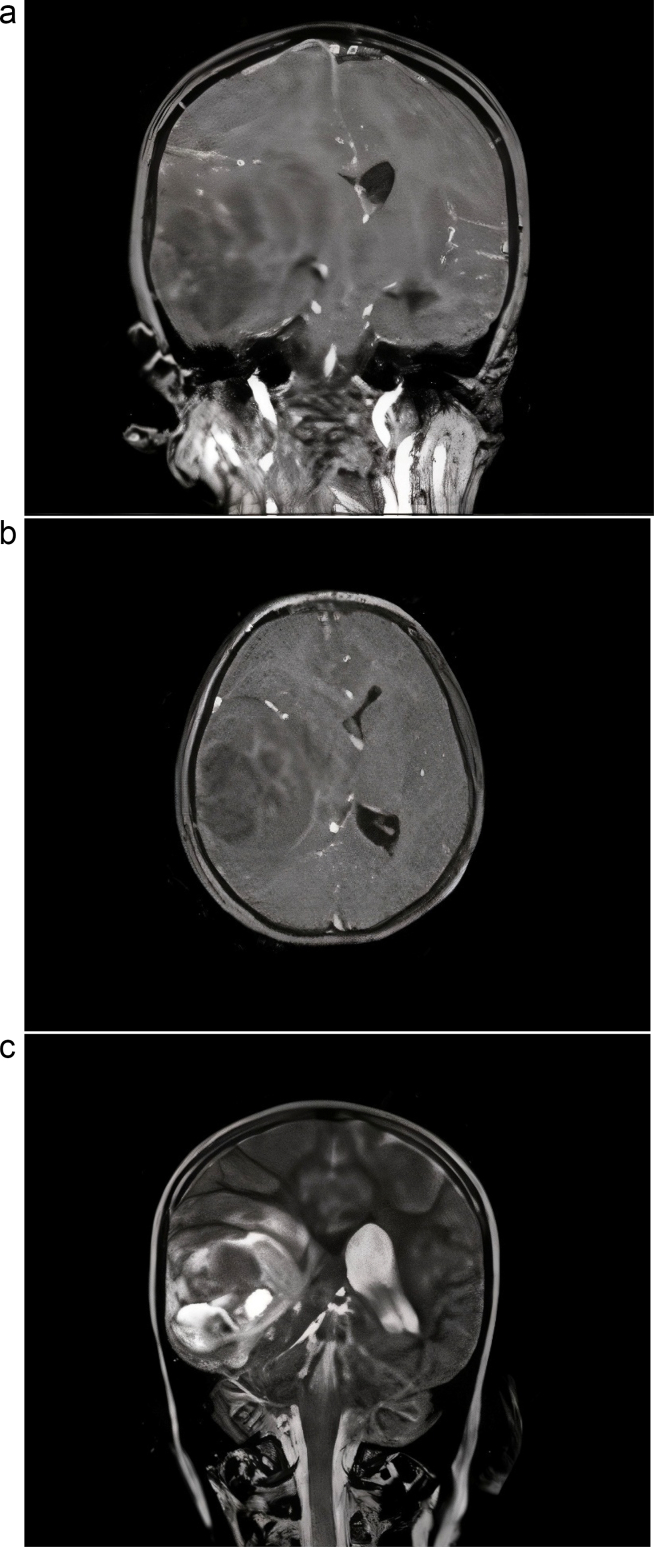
Magnetic resonance imaging at admission time. (a) T1 (coronal view) + gadolinium (contrast) injection. (b) T1 (axial view) + gadolinium (contrast) injection. (c) T2 (coronal view).
